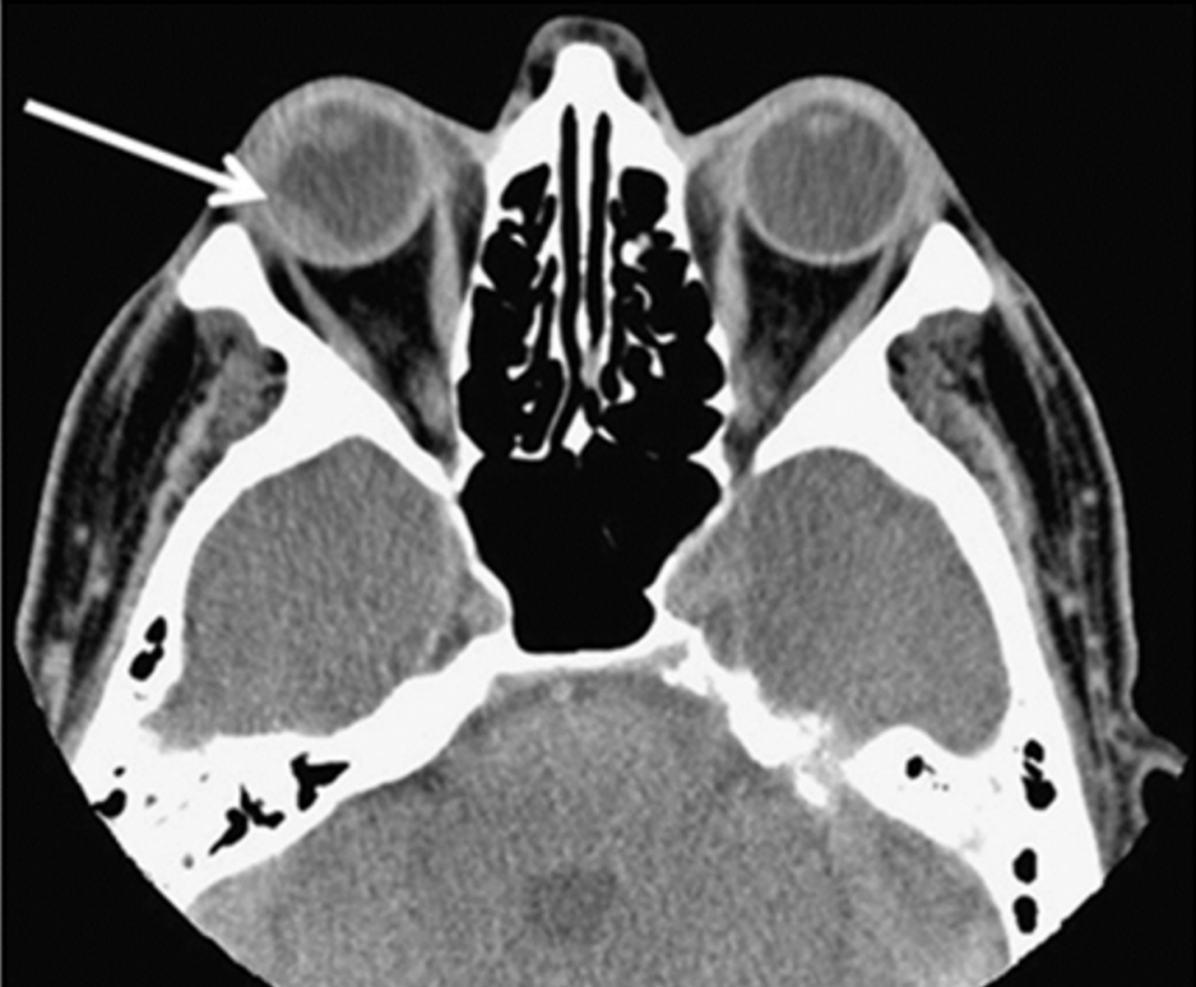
CT image demonstrating right ocular lymphoma lesion with extrascleral involvement CT scan showing a chorioretinal mass (arrow) in the right orbit, predominantly affecting the lateral and posterior parts of the globe, as well as transscleral extension laterally at the time of choroidal biopsy. (Reference: Mota, et al. [22]).
